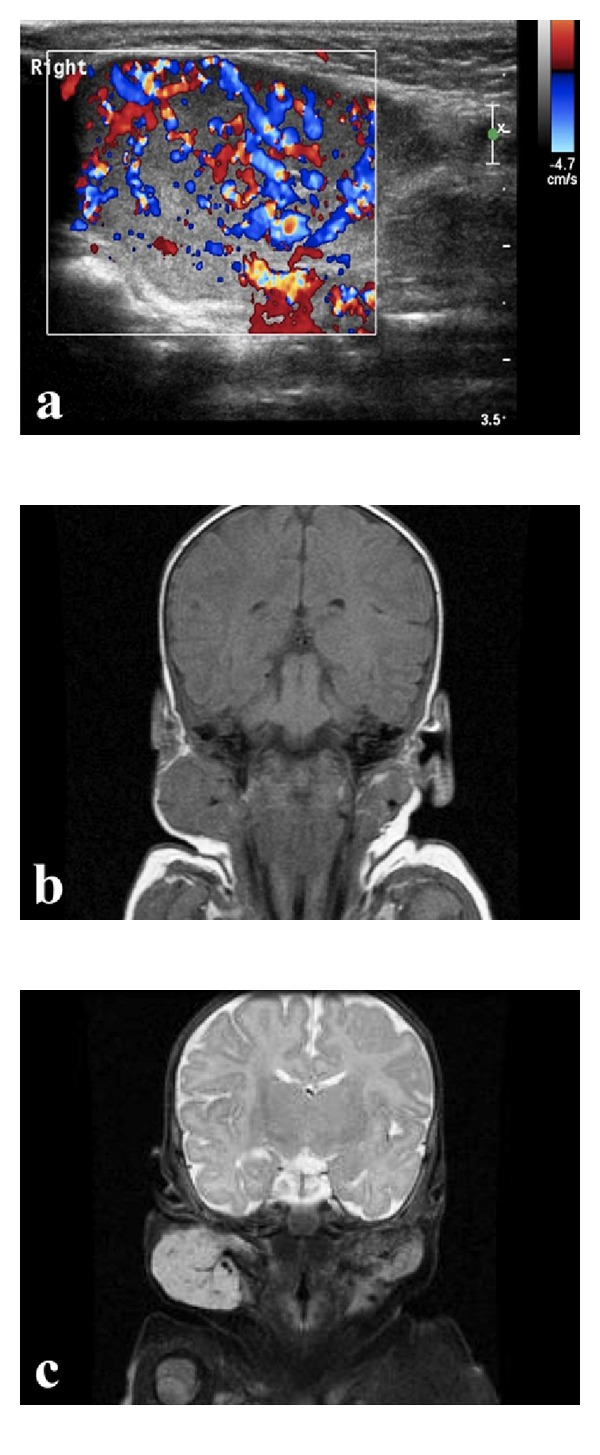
(a) Axial color Doppler sonogram of the right parotid showing an extremely hypervascular gland.(b) Coronal T1-weighted MR image showing a right parotid enlargement that is isointense to muscles. (c) T2-weighted MR image with fat saturation in coronal orientation showing a hyperintense right parotid mass with multiple flow voids.
